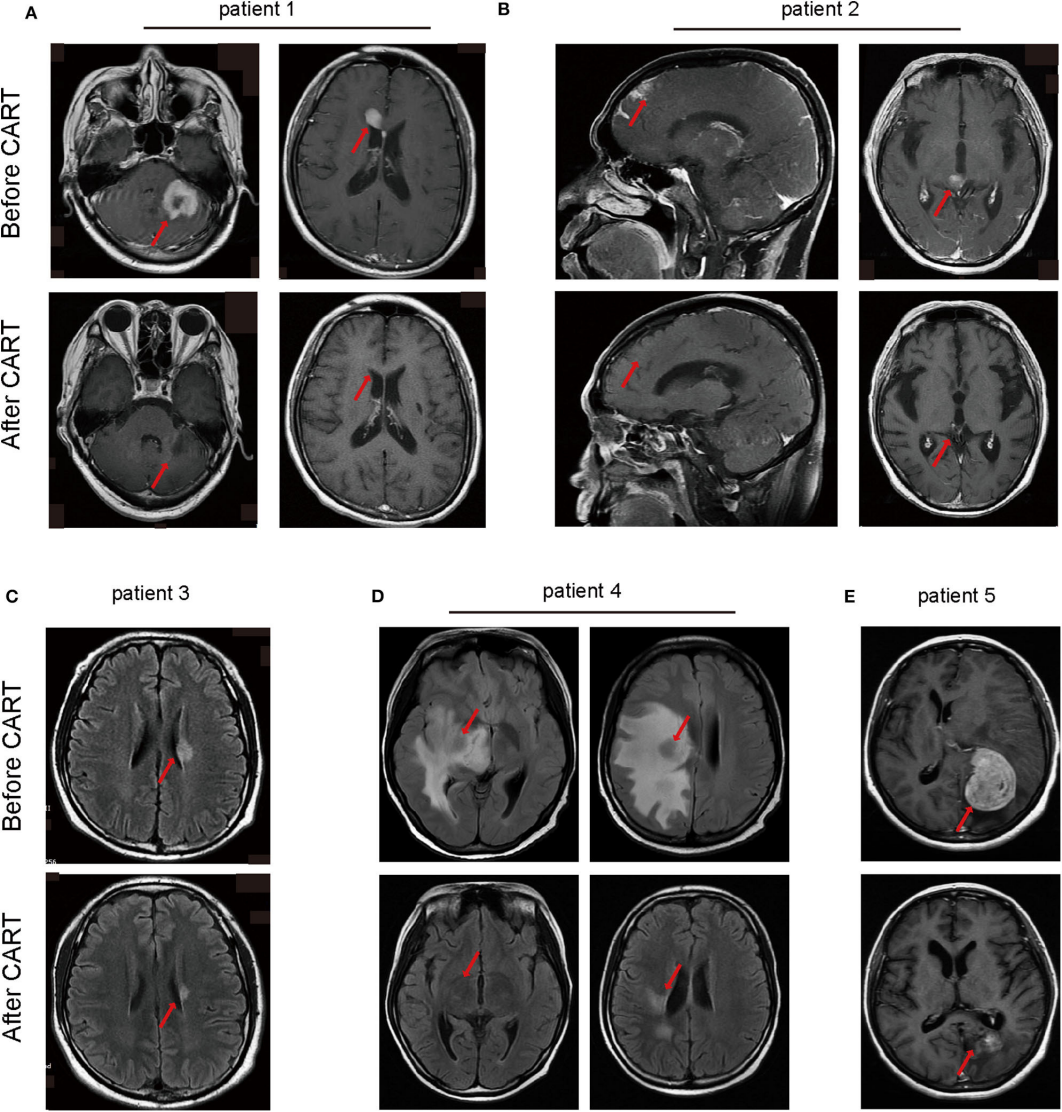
Head MRI imaging manifestations of lymphoma in CNS monitoring the therapeutic effects of CAR-T cell therapy of the five patients. (A) The main central invasion sites of patient 1 are left cerebellum and corpus callosum. (B) Invasion sites of patient 2 are frontal cortex and thalamus. (C) The central invasion site of patient 3 is left lateral ventricle. (D) The main central invasion sites of patient 4 are right basal ganglia, insula, frontal parietal temporal white matter, radiating crown, lateral ventricular trigonometry. (E) The invasion site of patient 5 is left occipital parietal lobe. MRI, magnetic resonance imaging.
